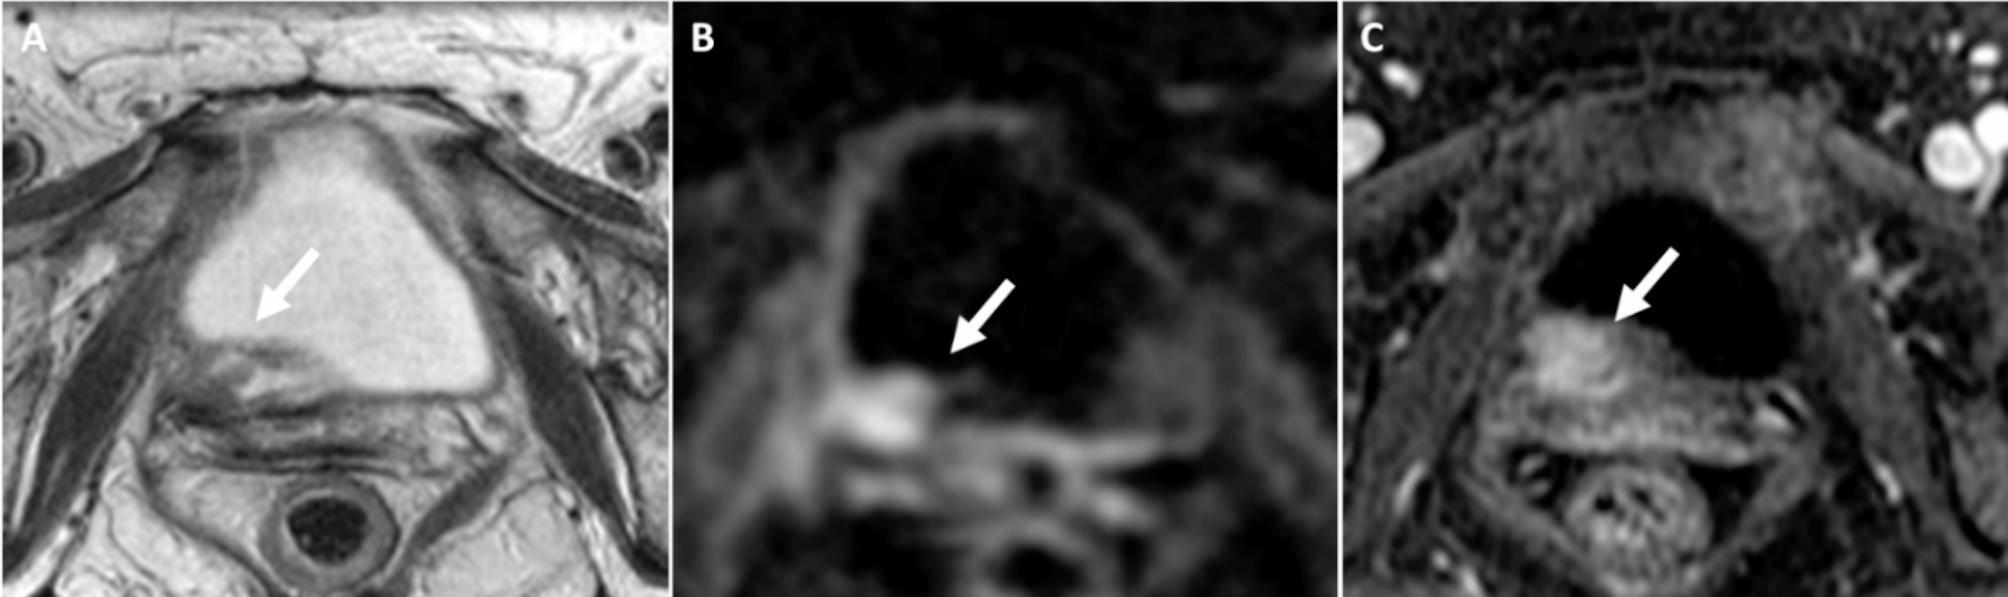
Fifty-five-year old woman with high grade muscle-invasive urothelial bladder cancer on transurethral resection. MRI performed after 4 cycles of neoadjuvant gemcitabine + carboplatin, showed an intermediate signal nodule (arrow) on T2-weighted imaging (A) with corresponding focal high signal on diffusion-weighed imaging (B) and early and strong enhancement on post-contrast MRI (C). At radical cystectomy, there was residual tumor with perivesical fat invasion (ypT3). Tumor recurred at the pelvic sidewall at 78 days after surgery and the patient subsequently died at 128 days after surgery
